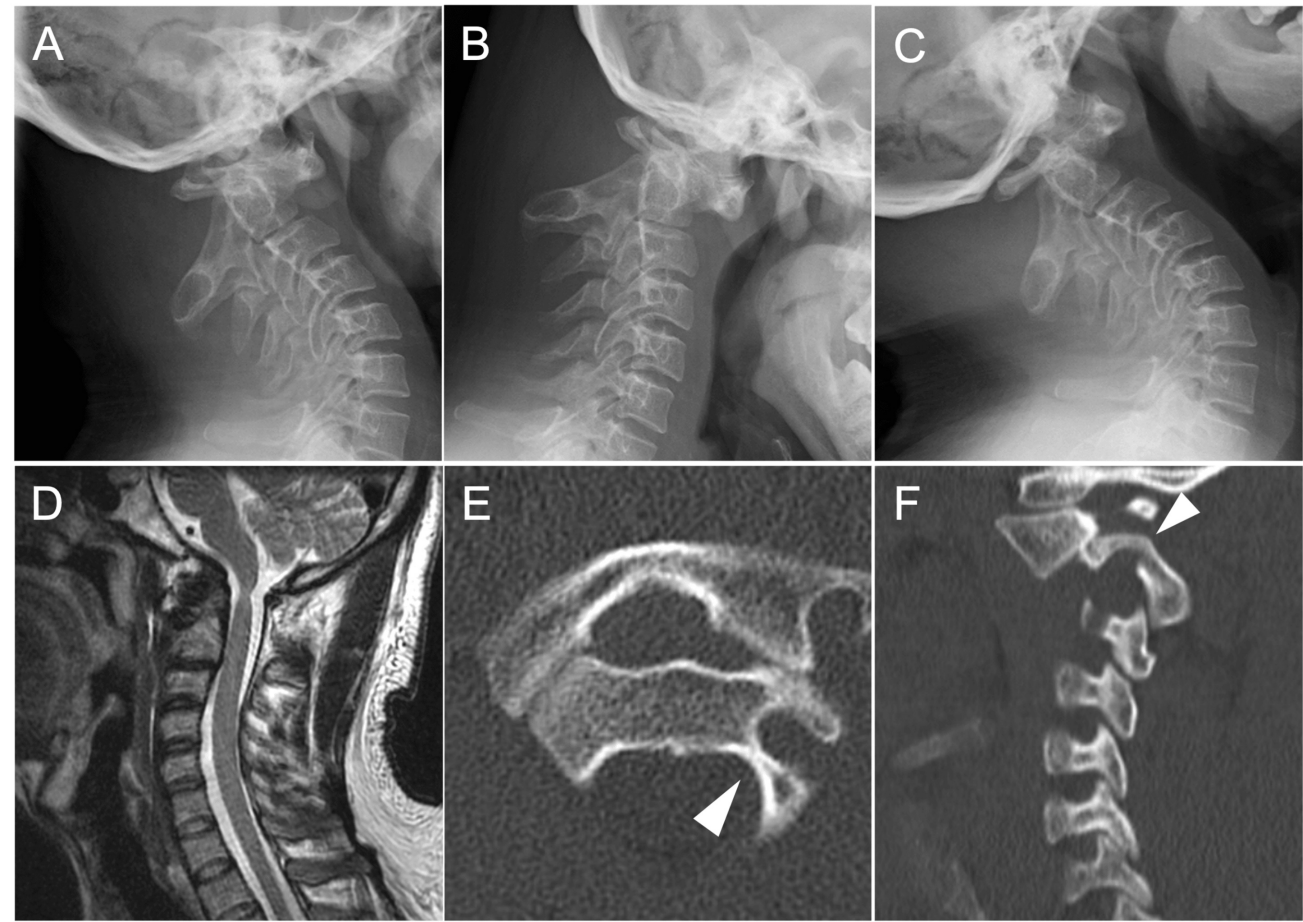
Preoperative imaging Lateral radiographs in neutral, flexion, and extension positions (A-C) demonstrate persistent atlantoaxial subluxation. T2-weighted sagittal magnetic resonance imaging (D) shows cervical cord atrophy with subtle intramedullary hyperintensity at C1-2. Contrast-enhanced computed tomography and computed tomography angiography (E, axial; F, sagittal) delineate a left high-riding vertebral artery and a markedly narrowed left C2 pedicle osseous corridor (white arrowhead) that precluded a left C2 pedicle screw trajectory.
